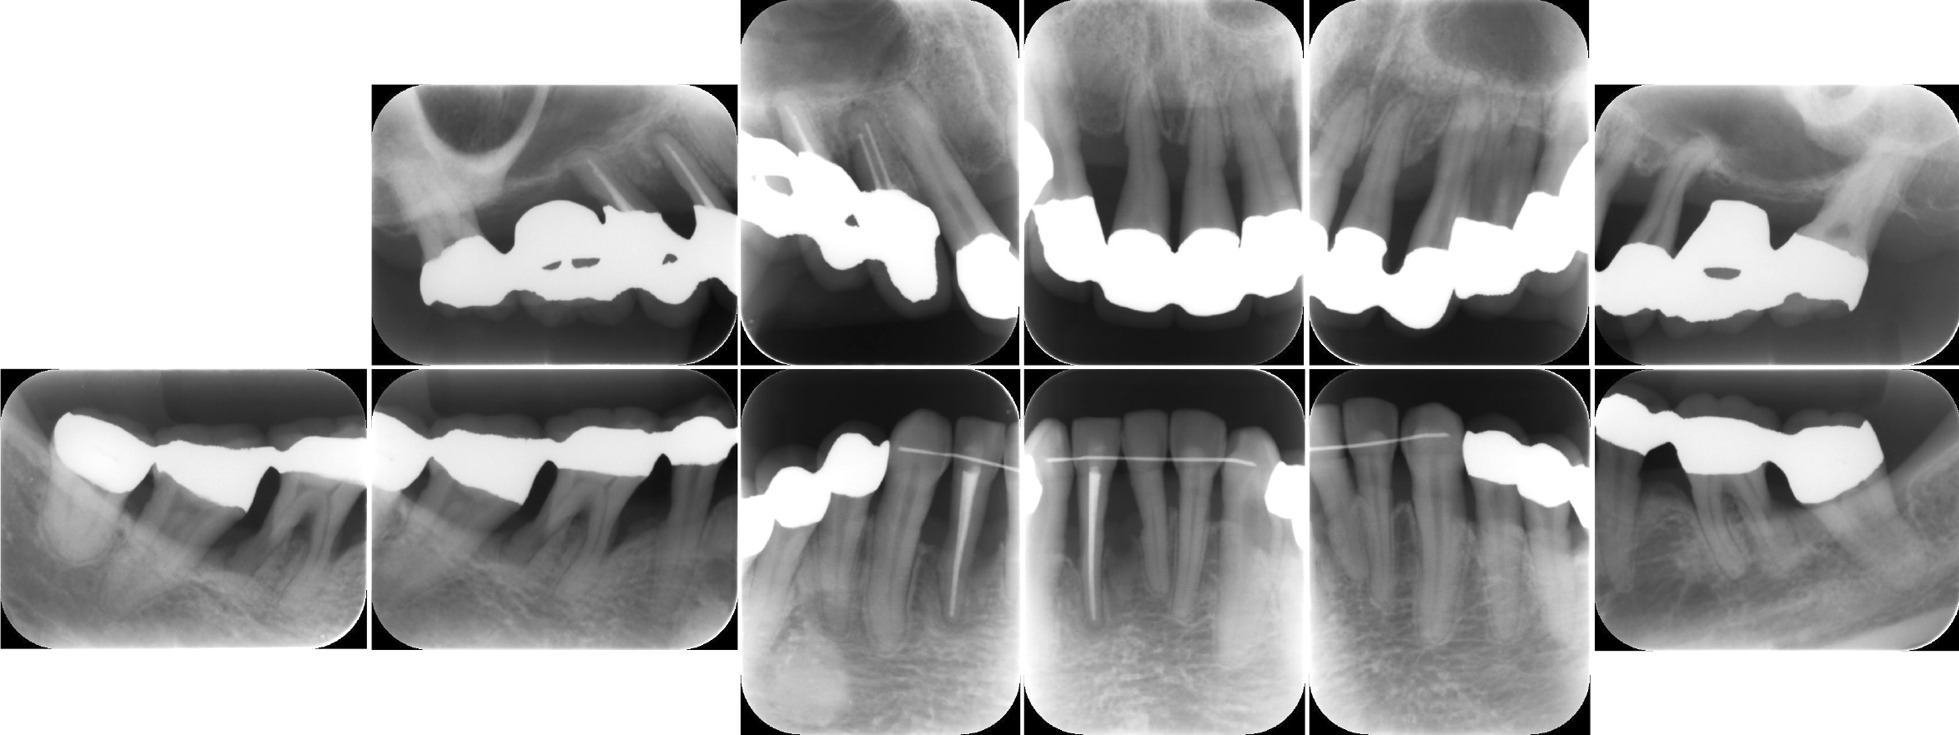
Dental periapical radiography. There are no signs indicating progression of bone resorption, and alveolar bone gain could be observed on the mesial aspect of tooth 17, the distal aspect of tooth 35, and the distal aspect of tooth 42 without bone grafting procedures
